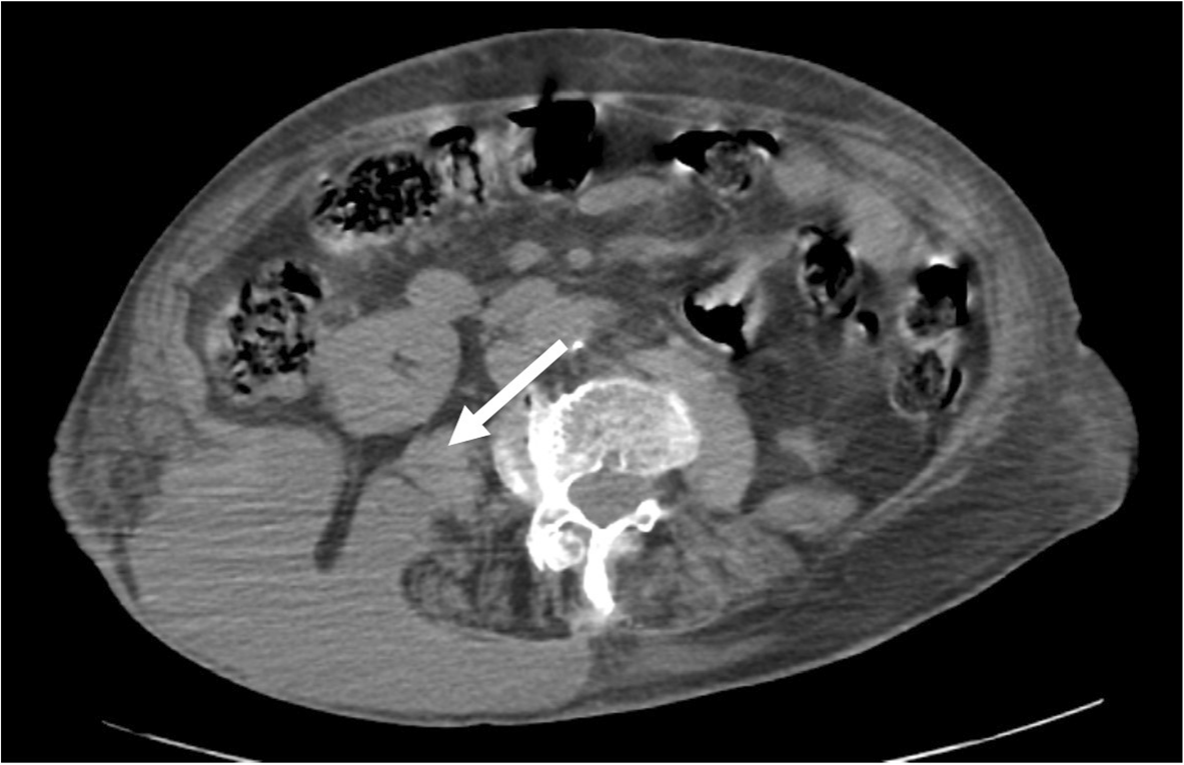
Axial computed tomographic scan without contrast media. Retroperitoneal abdominal collection with a large subcutaneous component in the lumbar region (see white arrow)
